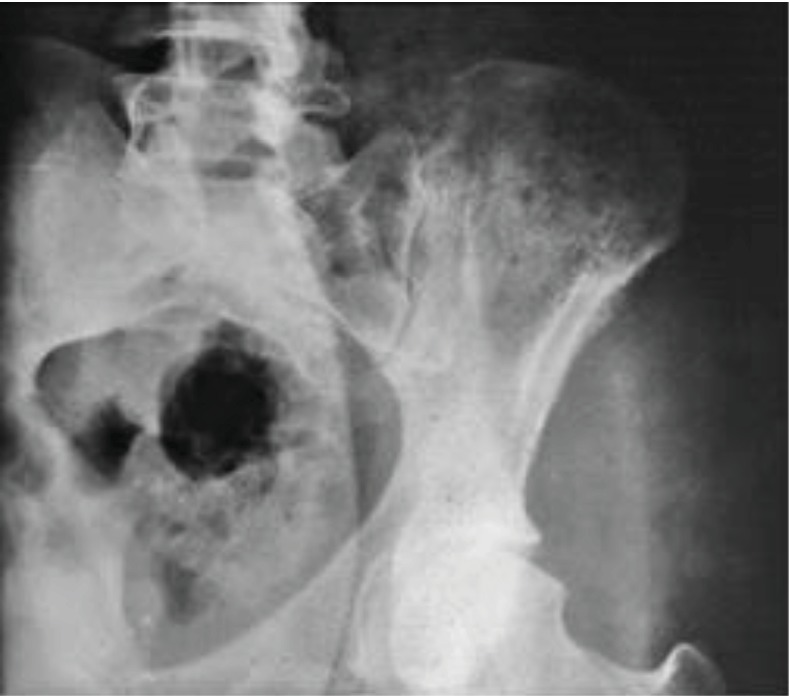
Iliac crest fibrosarcoma X-ray.
